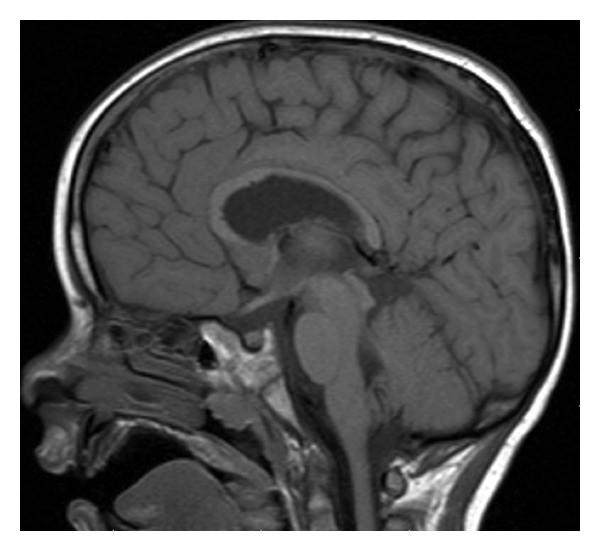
Preoperative MRI with apparent aqueductal obliteration.
